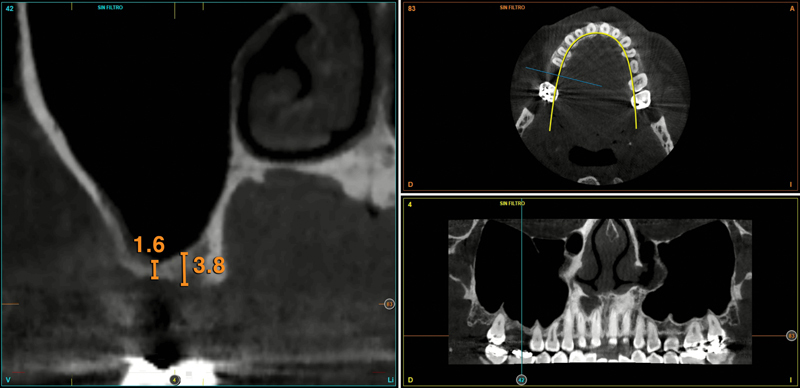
Cone-beam computed tomography image. Residual ridge measurements before the 4.5-length “temporary” implant placement. Note the discrepancy between palatal and buccal areas.
